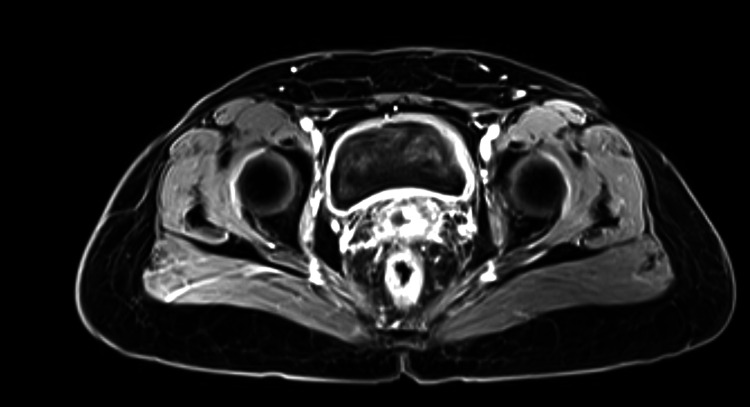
Follow-up CEMRI scan of the whole abdomen showing a complete response (axial section). CEMRI: contrast-enhanced magnetic resonance imaging
